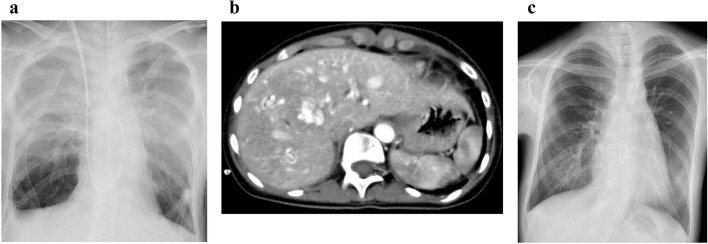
Postoperative chest X-ray radiography showed bilateral infiltrates due to ischemia–reperfusion injury that developed immediately after lung transplantation (a). Contrast-enhanced abdominal computed tomography showed the progression of hepatic arteriovenous malformations on postoperative day 30 (b). Chest X-ray radiography at discharge (c)
